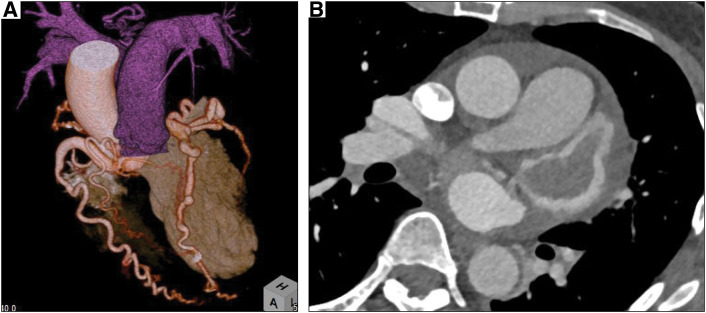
Computed tomographic reconstruction. The left coronary artery originated from the main pulmonary artery, with a mass in the left atrial appendage (A and B).
